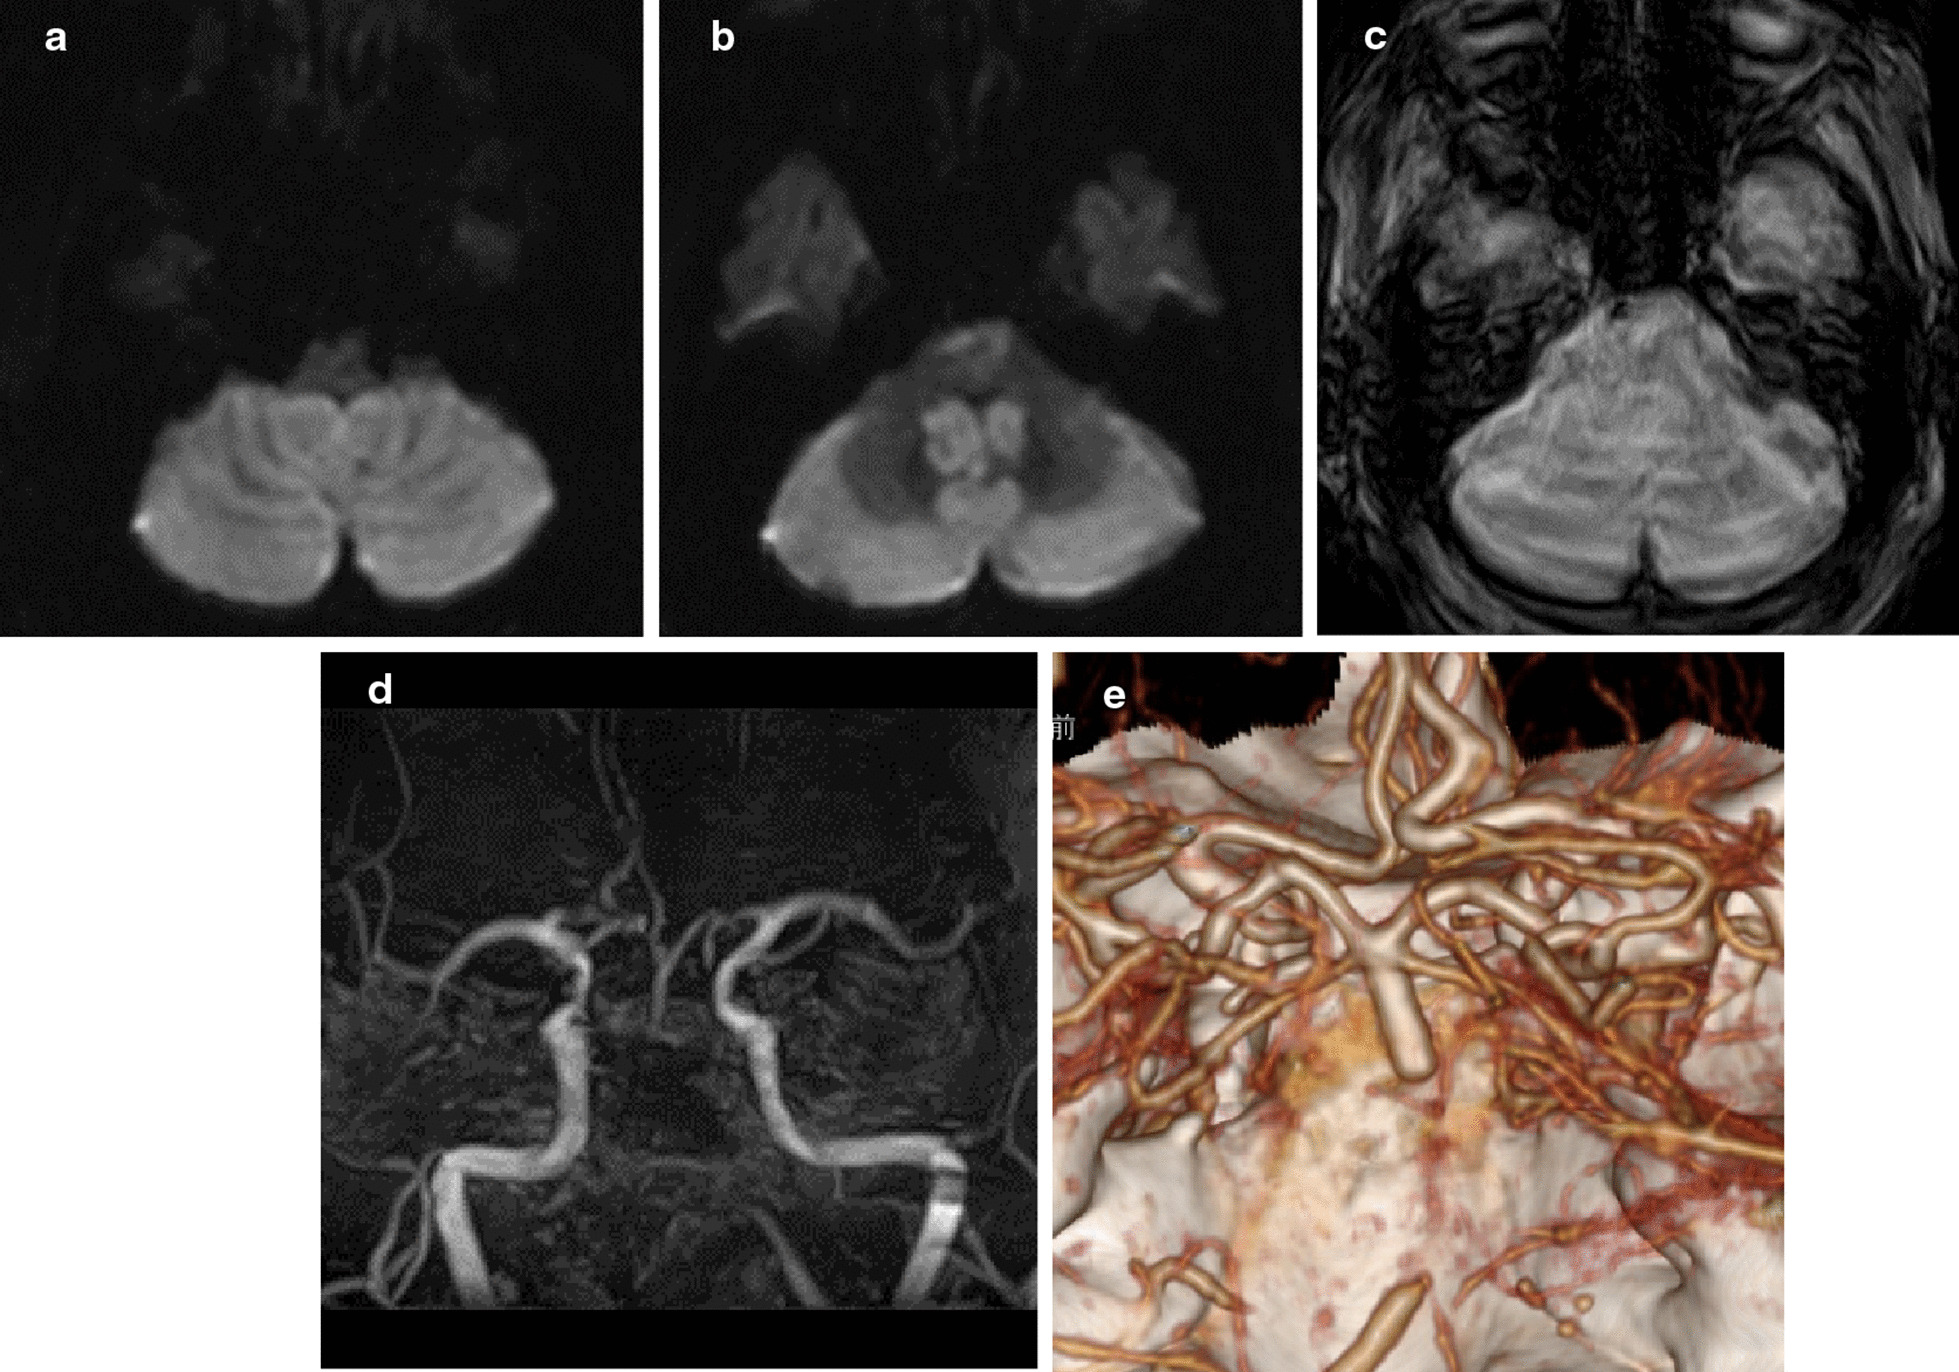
Diffusion-weighted brain MRI showing acute multifocal lesions involving the bilateral cerebellar hemispheres (a, b). The susceptibility vessel sign was noted in the middle portion of the basilar artery on the T2*-weighted image (c). The V3 segment of the right VA, the V4 segment of the left VA, and the lower third of the BA were occluded on MRA and 3D-CTA images (d, e)
